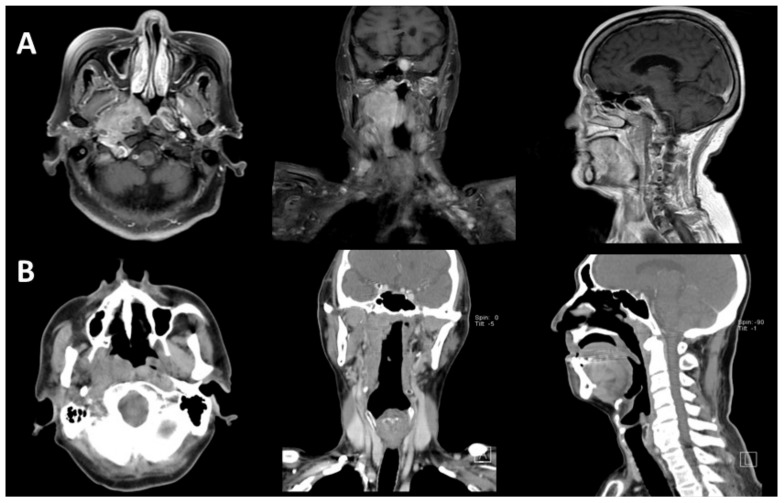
MRI and CT images of NP lymphomas. (A) An 85-year-old female diagnosed with NP DLBCL. The MRI revealed that the tumor involved the right NP and right parapharyngeal space. (B) A 73-year-old male diagnosed with NP NKTCL. The CT scan revealed that the tumor involved the right NP.
